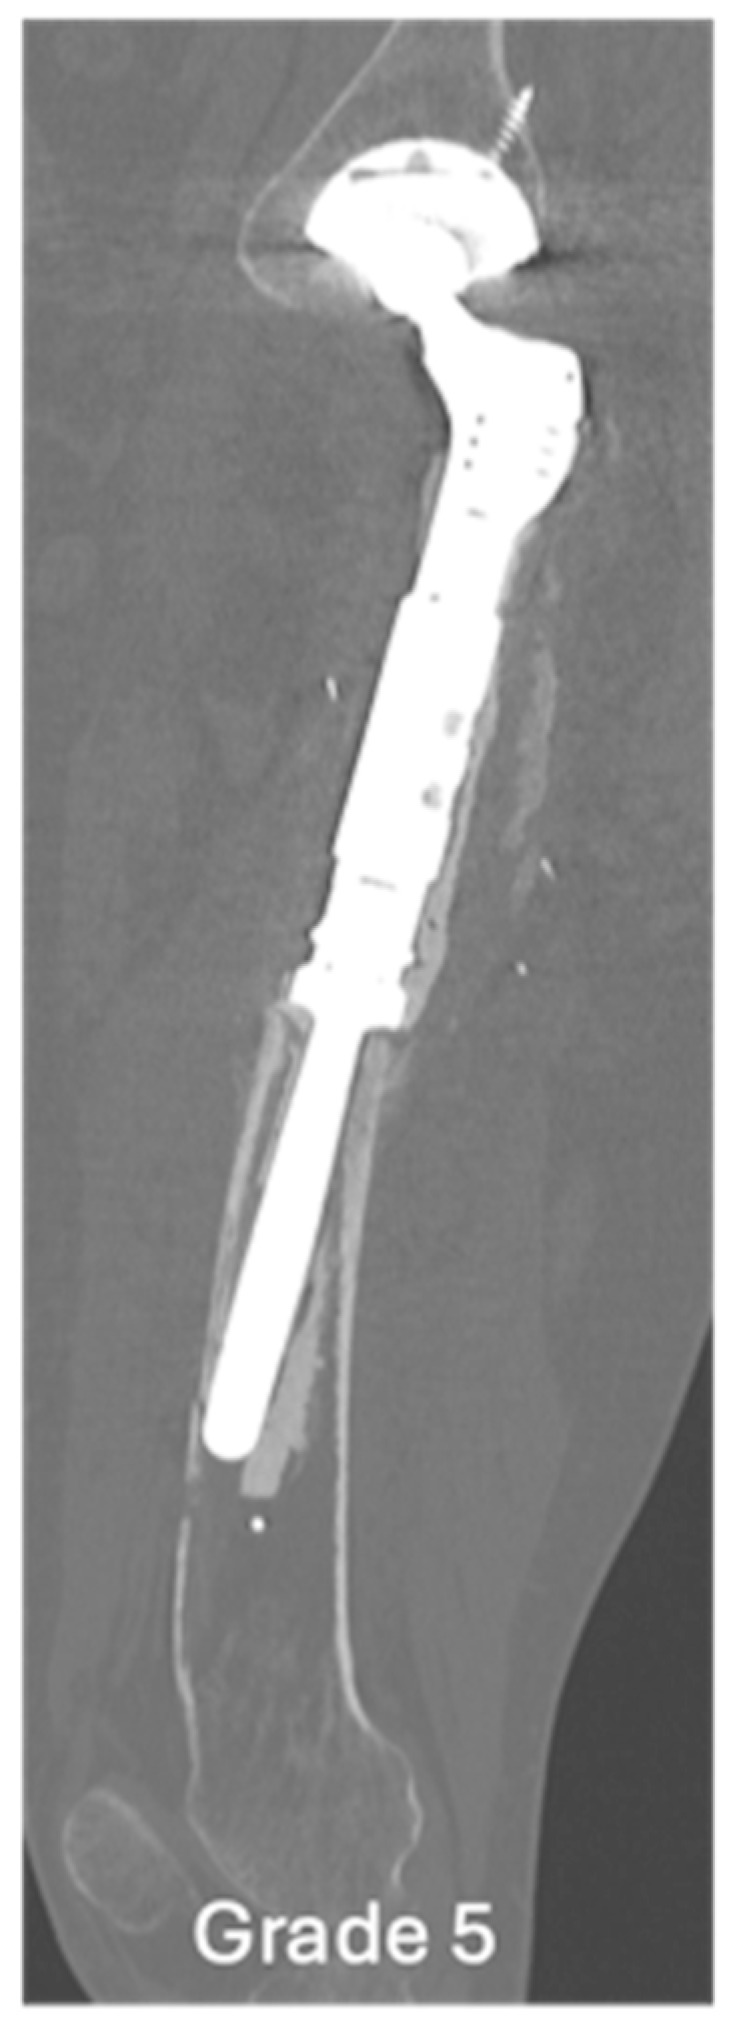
Sagittal CT showing grade 5 loosening of EPR, marked loosening of the femoral EPR with disruption of the anterior cortex.
